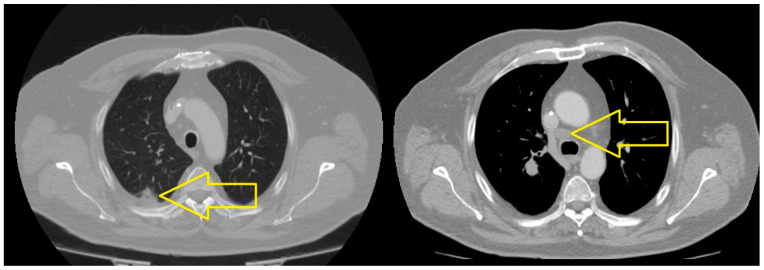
Chest CT scan (June 2023): A small nodular tissue lesion in the pulmonary parenchyma in the posterior segment of the right upper lobe with axial diameters of 18/15 mm and a dense tissular micro-nodular lesion located above the right posterior hilum with axial diameters of 17/15 mm. A small nodular lesion located in the right posterior apex with axial diameters of 9.6 mm The pulmonary lesions exhibit minimal regression from the previous CT scan. Several mediastinal adenopathies with diameters of up to 22 mm and in the right pulmonary hilum with diameters of up to 30/26 mm.
